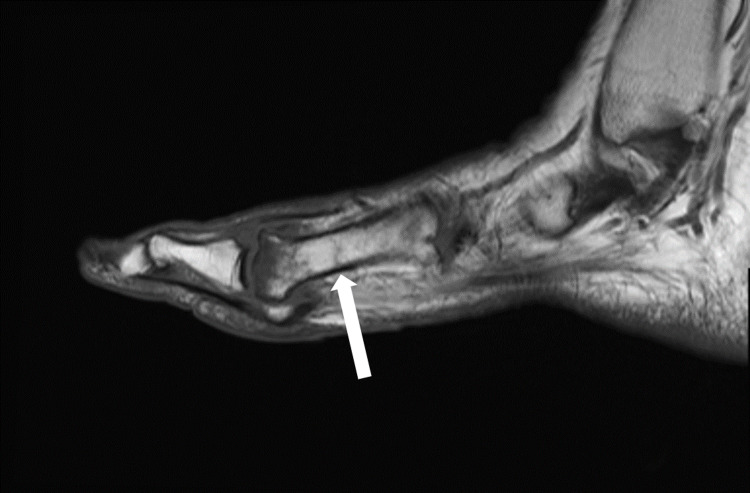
T1-weighted sagittal MRI of the right foot demonstrating a first metatarsal shaft stress fracture (arrow).
